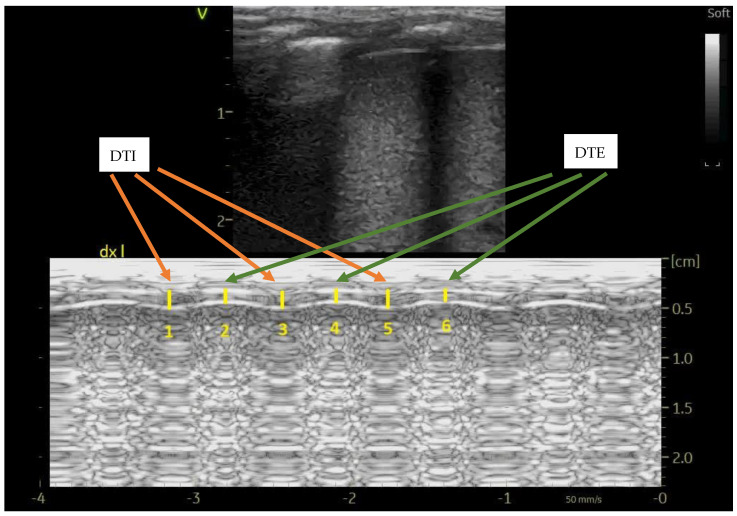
Diaphragm ultrasound in M-mode showing the DTI (average value of measures number 1, 3 and 5) and DTE measurements (average value of measures number 2, 4 and 6). The probe was longitudinally applied on the right mid-axillary line (diaphragm apposition zone).
